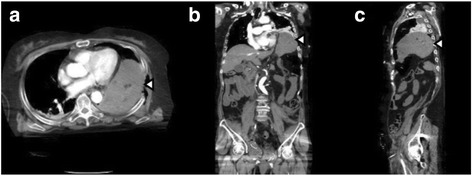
a–c This computed tomography showed herniation of the stomach through the diaphragm rupture after temporarily closing the thoracotomy incision
